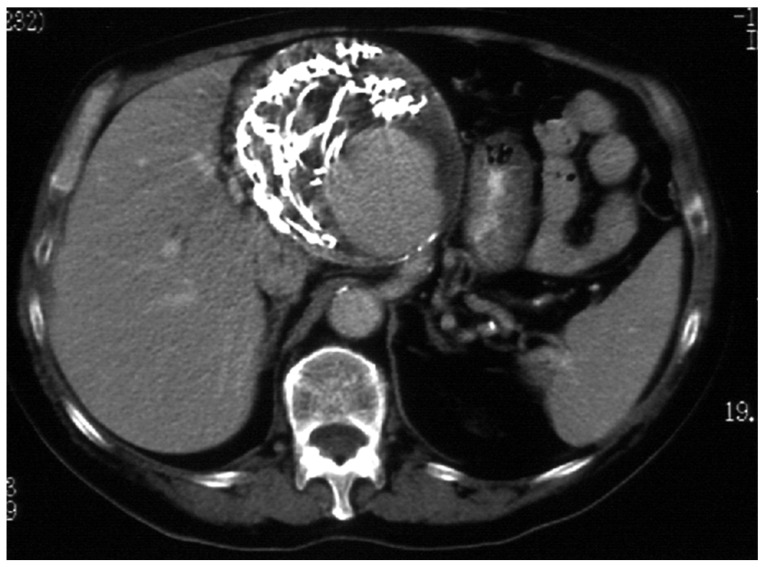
Post-operative CTA following common hepatic artery embolization.
